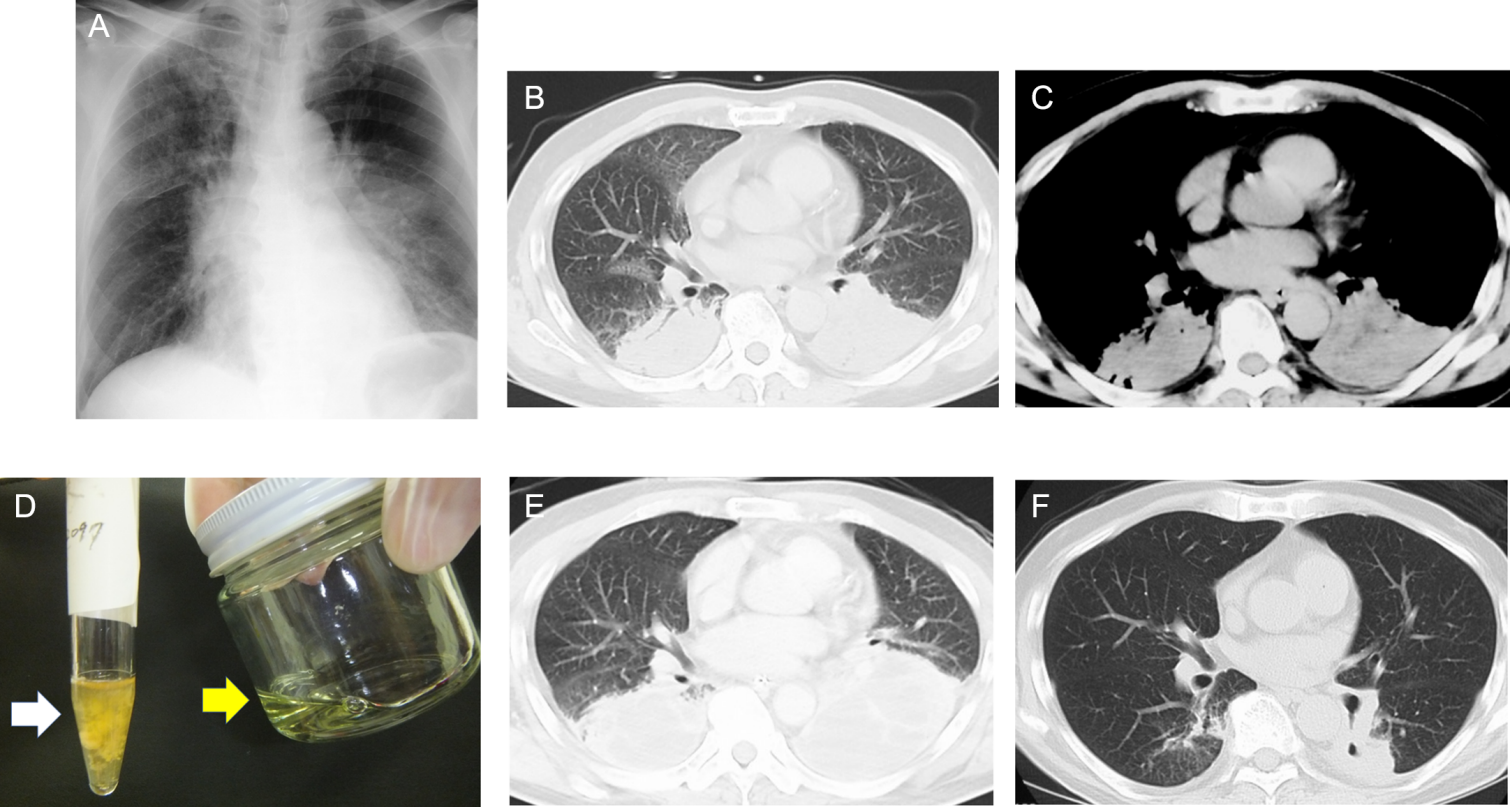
(A) Chest X‐ray showing the increment of bilateral hilar shadow. (B) Thoracic computed tomography (CT) showing infiltrative shadows in both lower lobes. (C) Thoracic CT showing low‐density area in infiltrative shadows in both lower lung lobes using a mediastinal window. (D) The collected fluid using bronchoalveolar lavage (white arrow) resembling wood preservative (yellow arrow). (E) Thoracic CT at 2 weeks after the first CT, showing worsening of infiltration of both lower lobes. (F) Radiological improvement was noted a month after the first CT.
